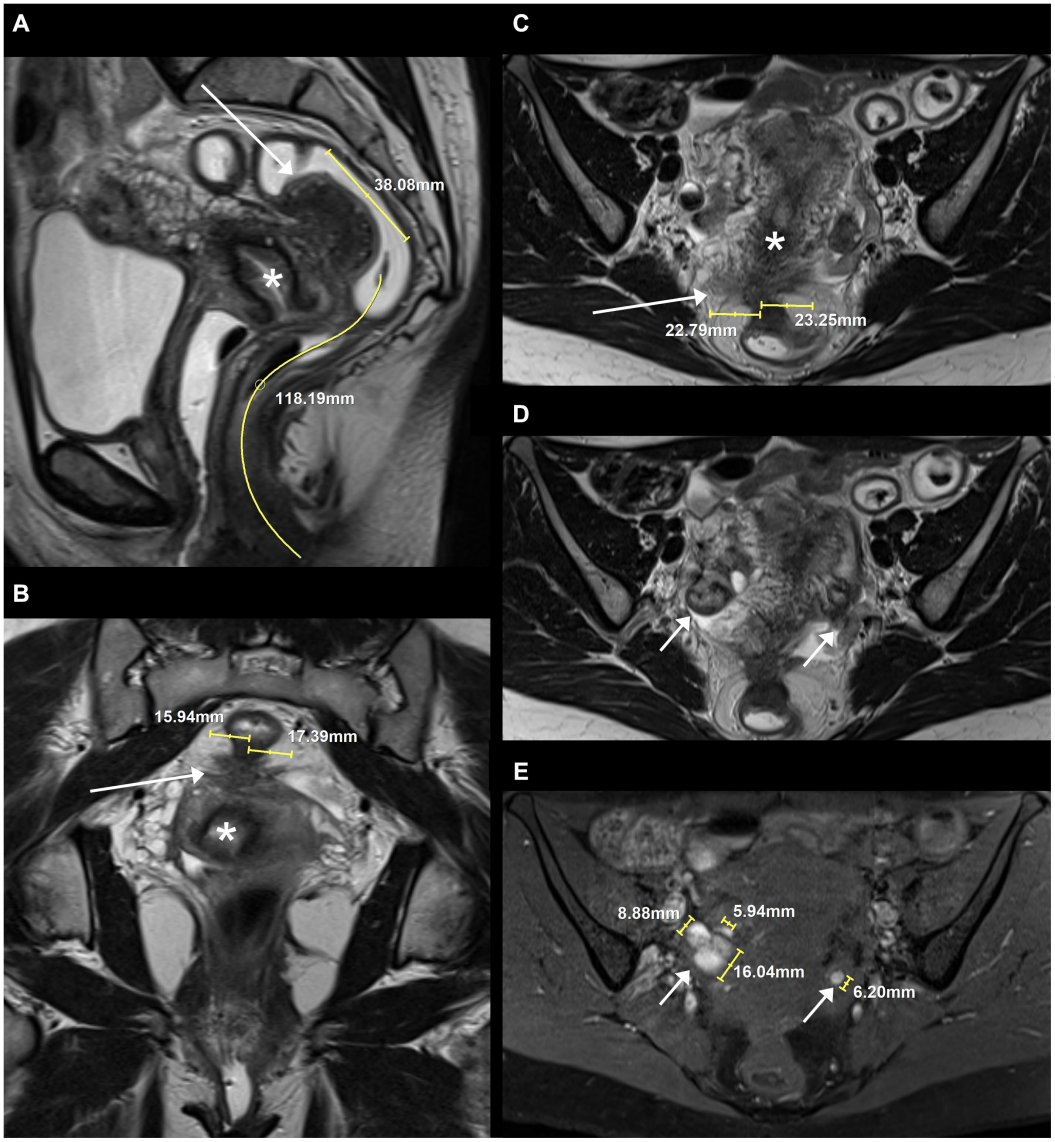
Example of typical deep infiltrating endometriosis (DIE), focal adenomyosis of the outer myometrium and endometriomas on MRI, categorized by two readers as #Enzian(m) O1/2, A2, B2/2, C3, FA and by one reader as #Enzian(m) O1/1, A2, B2/2, C3, FA (due to a borderline summed size of endometriomas on the right side between O1 and O2): (A) Sagittal, (B) coronal and (C) axial T2 FSE (fast spin echo) showing a hypointense mass containing hyperintense foci (long arrows) with extension to rectum, vaginal vault, parametria, and posterior outer myometrium. (D) Axial T2 FSE and (E) axial fat suppressed T1 FSE demonstrating characteristic bilateral endometriomas with T1w-hyperintensity and T2w-hypointensity (short arrows). Asterisks: cervix uteri.
